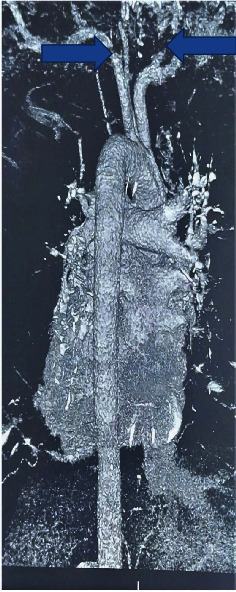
Computed tomography and pulmonary angiography of the chest in the index patient showing near complete occlusion of both right and left pulmonary arteries.
